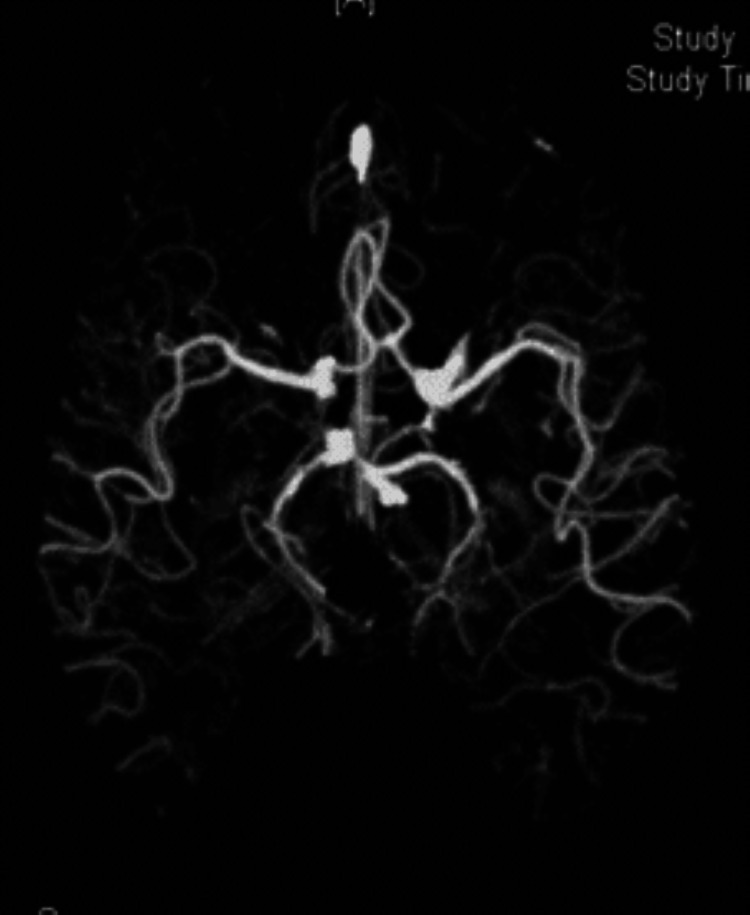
CTA showing opacification of the anterior and posterior circulation with no visible large or medium diameter vessel occlusions
